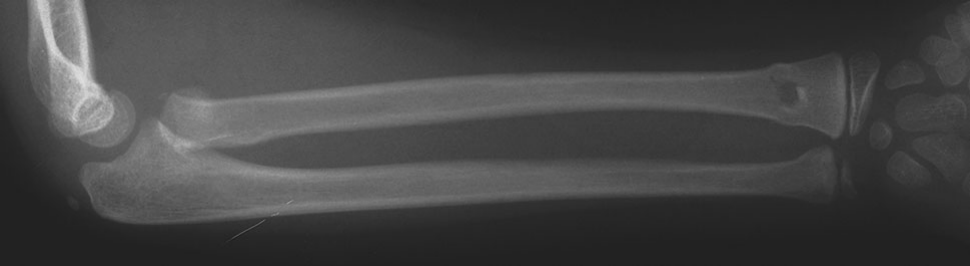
Radiograph of an 8-year-old boy with a type I segmental injury of the radius. It consisted of a displaced Salter-Harris type II physeal injury of the proximal radius associated with an undisplaced fracture of the distal part of the radius and ulna
